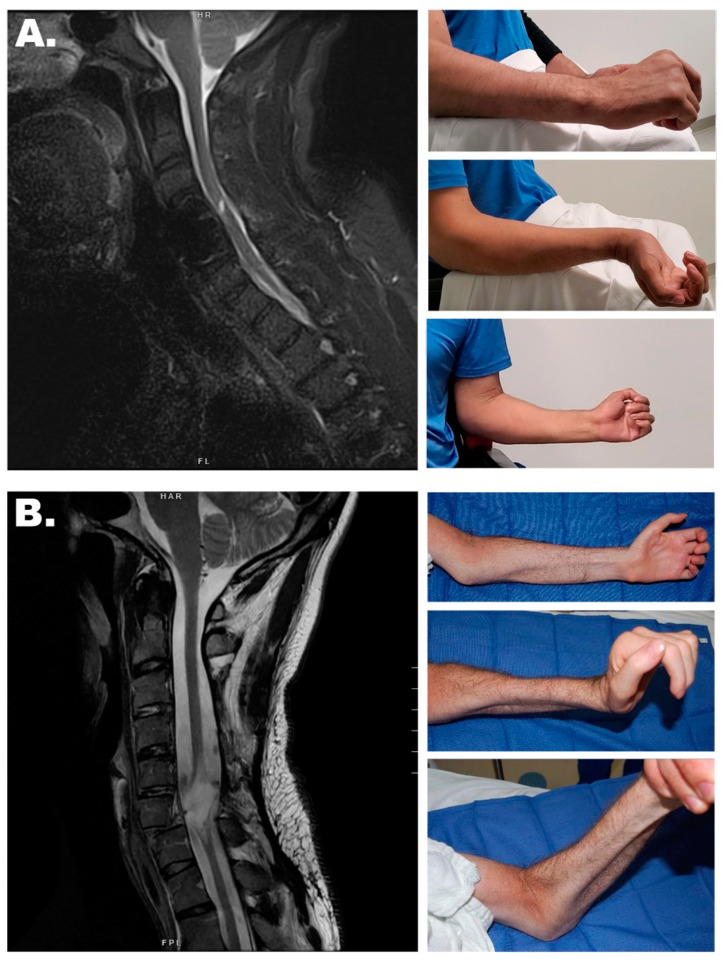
Signs of Denervation Atrophy. (A) This cervical MRI demonstrates a limited spinal cord injury. In spite of the fact that this was a C6 motor complete SCI, there is very little loss of gray matter and the paralyzed muscles of the forearm and hand remain innervated and therefore maintain substantial muscle bulk and tone. (B) In this C7 motor complete SCI, the cervical MRI shows extensive destruction of spinal cord tissue. This tissue contains the SMNs and their destruction results in Wallerian degeneration of their associated peripheral axons. This LMN injury results in severe atrophy of the associated muscles in the forearm and hand.
